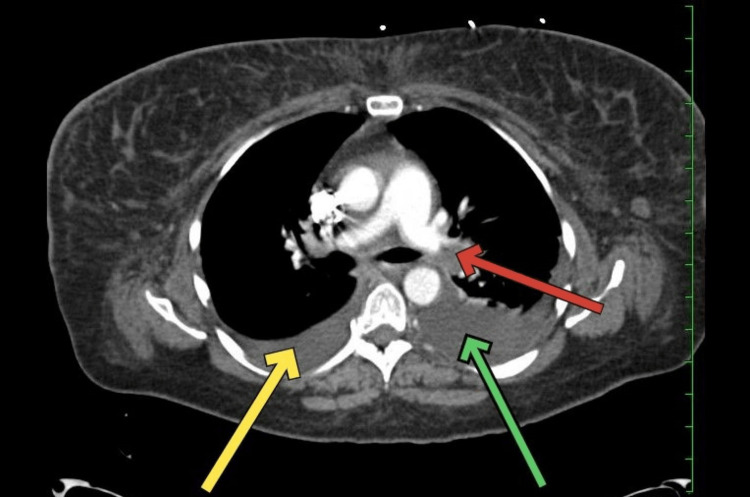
CT pulmonary angiography showing a pulmonary embolus CT pulmonary angiography showing a red arrow pointing at a pulmonary embolus in the left pulmonary artery, a yellow arrow pointing at the right pleural effusion, and a green arrow pointing at the left pleural effusion CT: computed tomography
